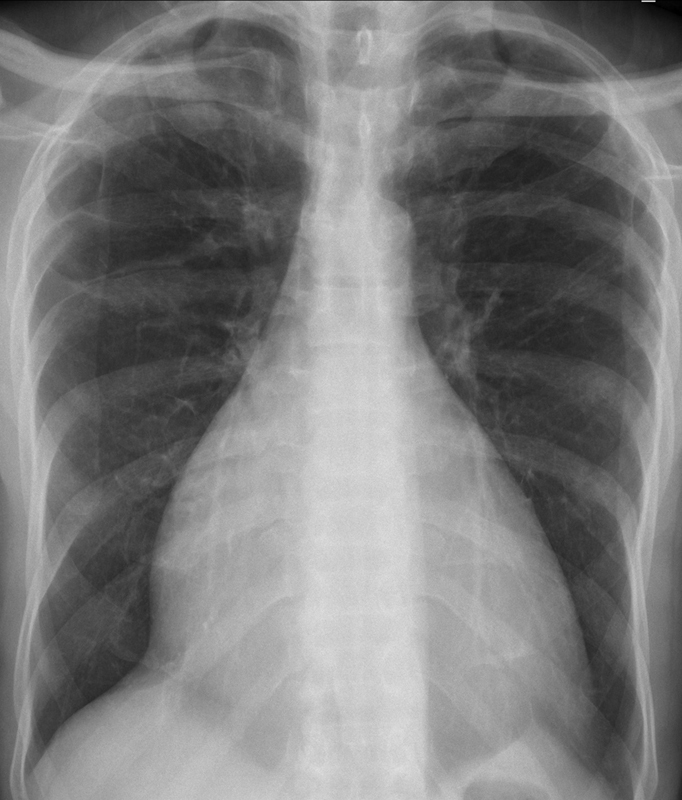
Chest X-ray showing cardiomegaly and normal pulmonary parenchyma.
